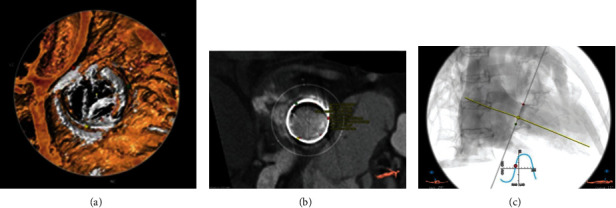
Cardiac computed tomography images: distribution of severe bioprosthetic leaflet calcification (a). Inner diameter of the bioprosthetic valve (26.3 mm) (b). The best projection angles (c).
